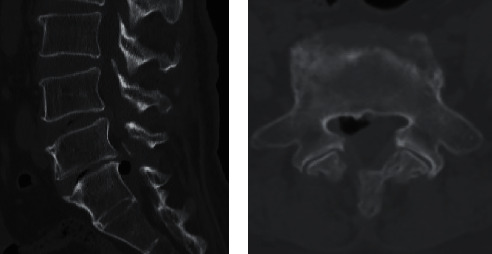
CT reveals a single large epidural gas bubble mimicking disc herniation on MRI as shown in axial (a) and sagittal (b) cuts. A coexisting vacuum disc phenomenon indicates the presumed origin of the gas bubble.
